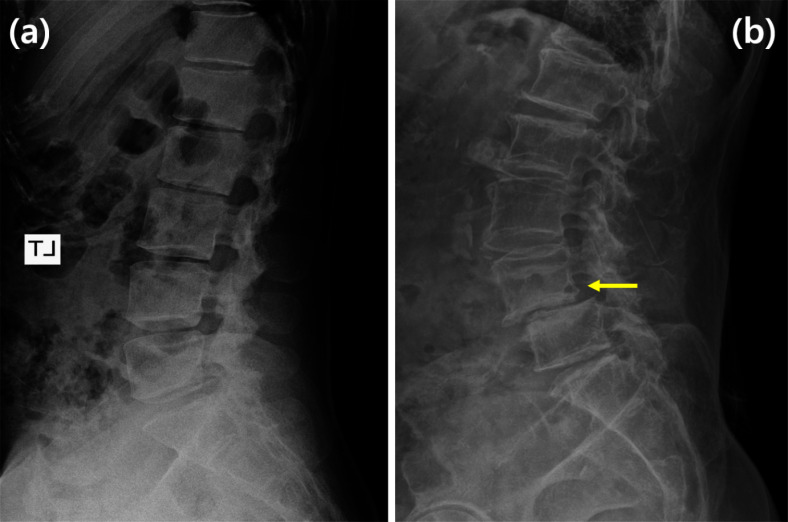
Examples of X-ray images: a normal condition and b spondylolisthesis
